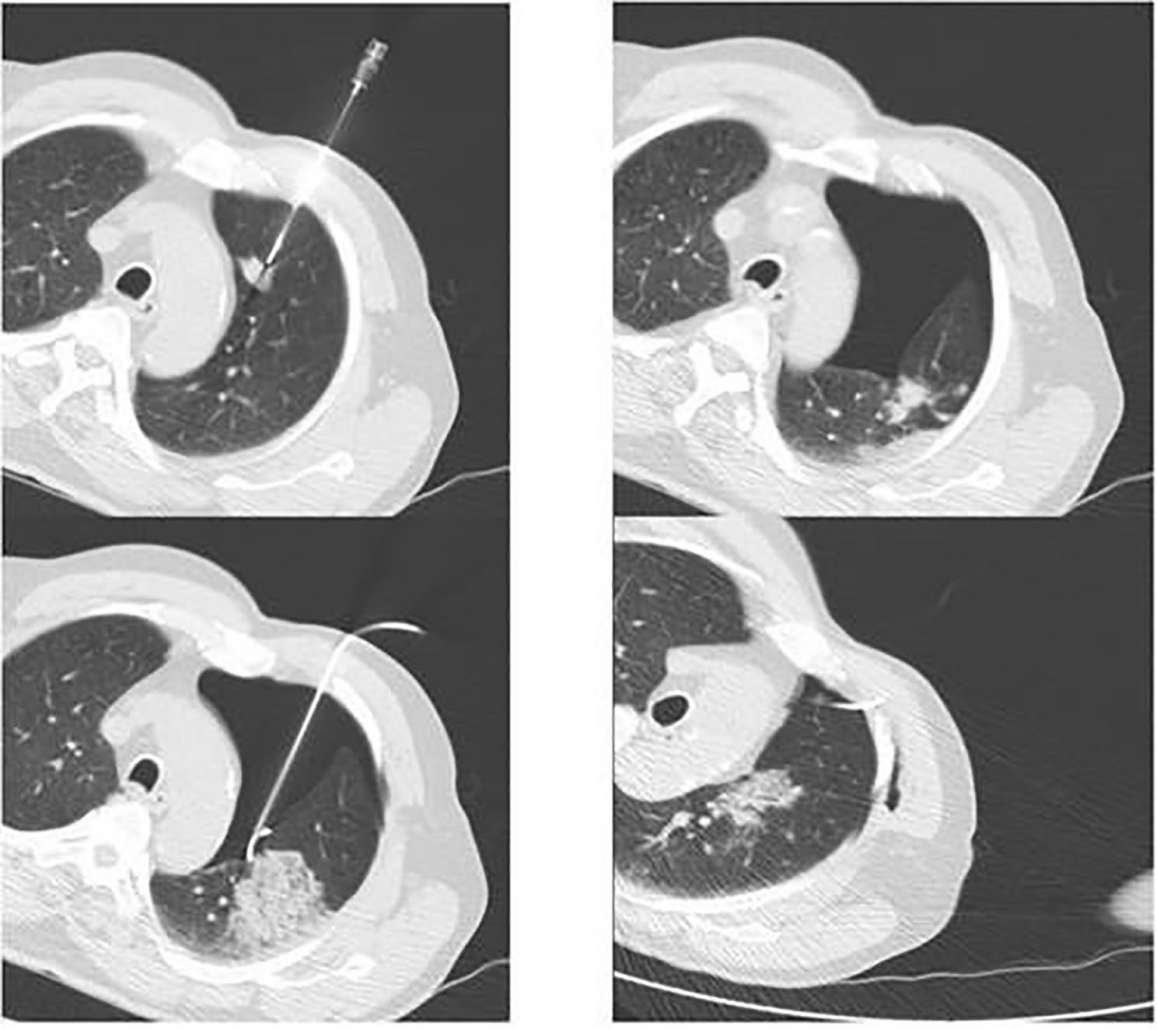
CT guided transthoracic needle biopsy from a lesion in left lungs upper lobe followed by pneumothorax and parenchymal hemorrhage, insertion of a pigtail catheter and aspiration of air
